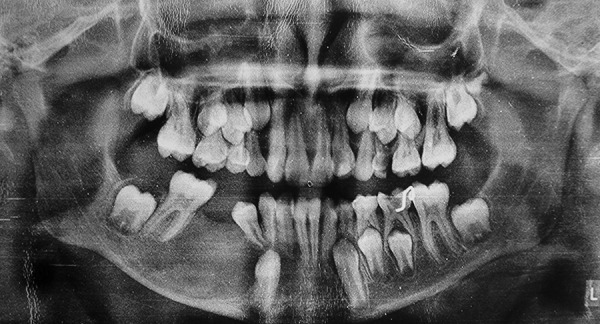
Postoperative panoramic X-ray at 6 months
